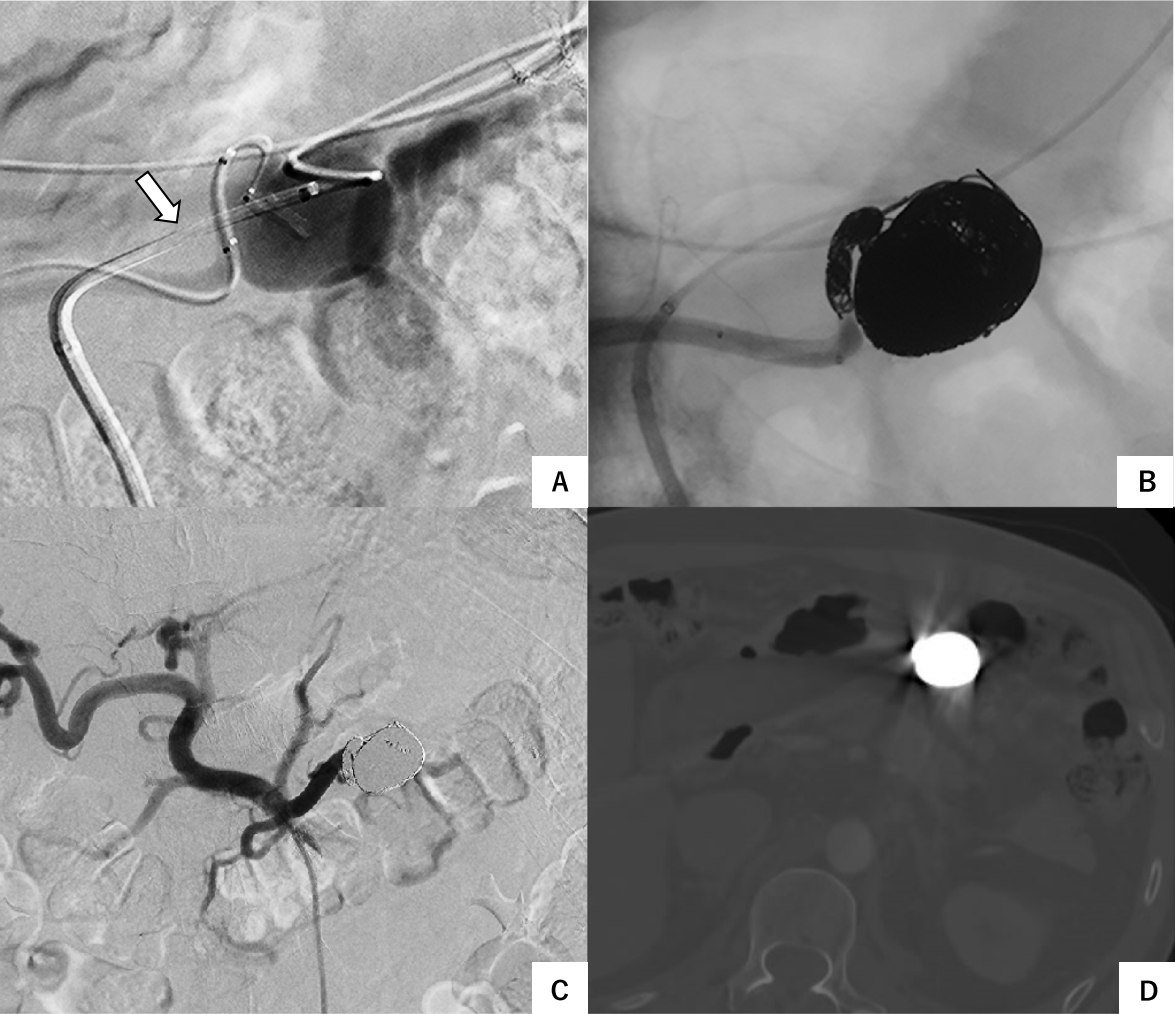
A The 4-axis catheter system (white arrow) was used due to the long and tortuous distance from the celiac artery. B The sac and the end of the feeding artery were embolized with microcoils. C Celiac arteriography after embolization. D Contrast-enhanced CT 6 months after embolization showed complete occlusion
